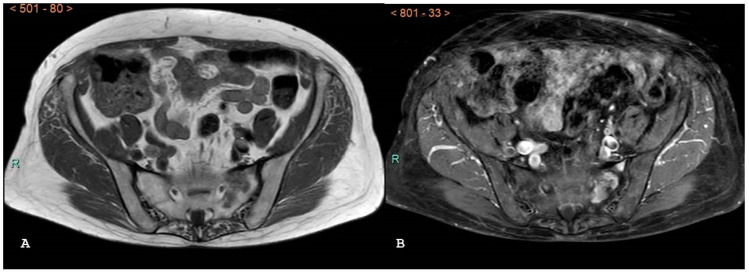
Axial T1-weighted (A) and axial T1 fat-saturated after gadolinium contrast (B) magnetic resonance imaging sequences demonstrate a metastatic lesion in the left sacral ala, adjacent to the sacroiliac joint, in a 70-yr-old woman with myeloma. The lesion appears hypointense in the T1-weighted sequence and has inhomogeneous contrastographic enhancement prevalent at the periphery related to the localization of disease in a nonactive phase.
